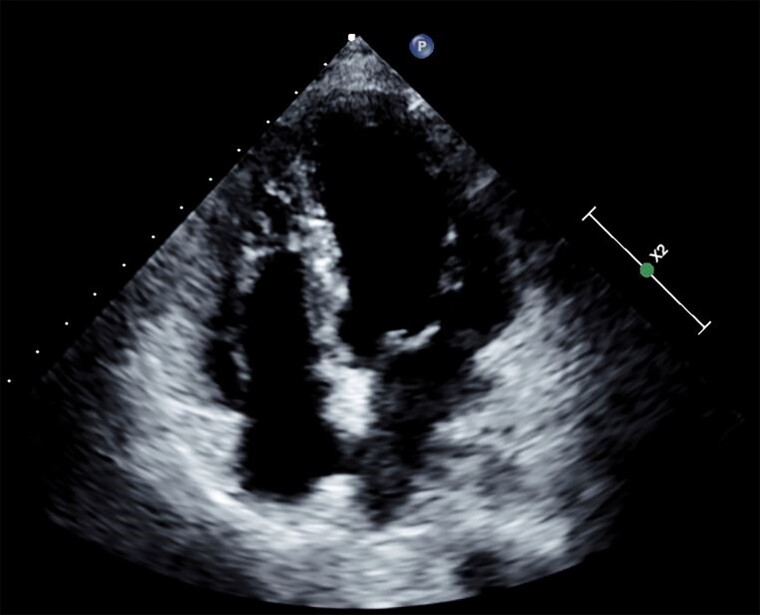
Transthoracic echocardiography (apical four-chamber view) showing complete resolution of pericardial effusion along with normalization of the right ventricular size.
